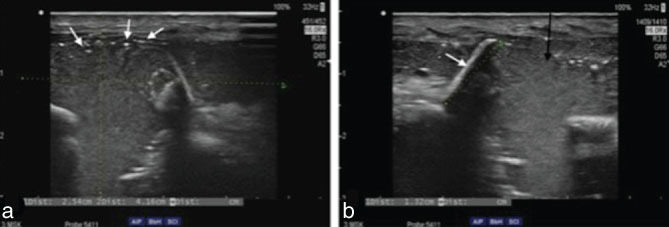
Ultrasonography of the supraclavicular region. (a) Subcutaneous hyperechoic, complex fluid collection extending above the right clavicle and between the clavicle fragments, with the presence of hyperechoic gas bubbles (white arrows). (b) A free fragment of the clavicle cortical bone (white arrow) surrounded with thick, hyperechoic fluid collection (black arrow).
